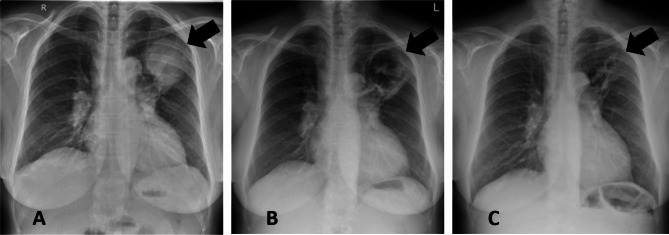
Evolution of chest imaging in a patient affected by Legionnaires’ Disease lung abscess. Evolution over time of chest X-ray of the second reported case, from right to left respectively (subpanel A, B, C). The lung lesion is underlined with a black arrow. A (left): left apical consolidation; B (middle): evolution of the consolidation into cavitation; C (right): partially resolution with shrinking of the lesion
